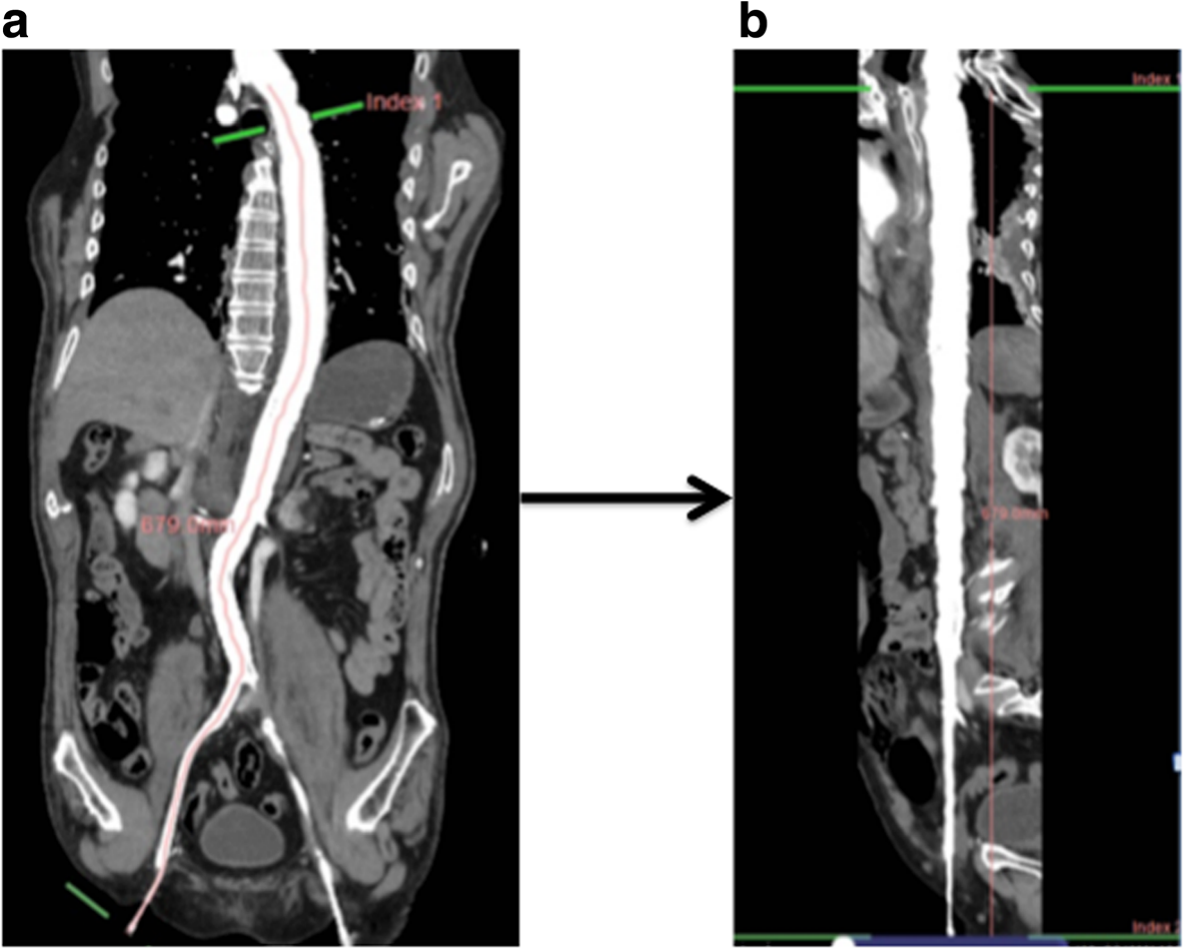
How to measure the blood vessel length using Ziostation 2 PLUS. a We plotted the center point of the contrasted vascular lumen from the common femoral artery to the blood vessel cross section at the tip of REBOA at the horizontal disconnection of CT. b We reconstructed meandering blood vessels approximately linearly and measured the blood vessel length
